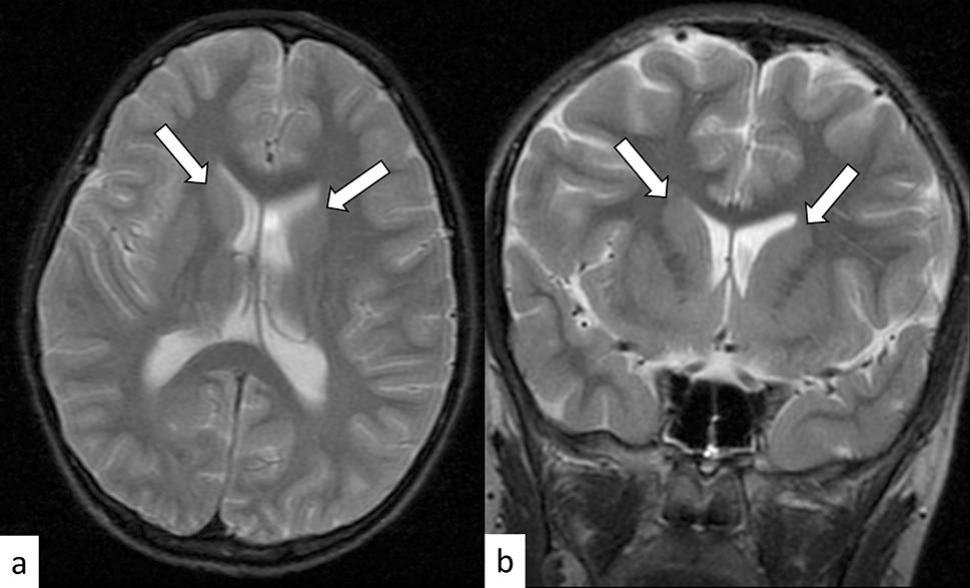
A 4-year-old girl with fragile X syndrome. T2-weighted axial a and coronal b images show slightly enlarged heads of the caudate nuclei (arrows)
